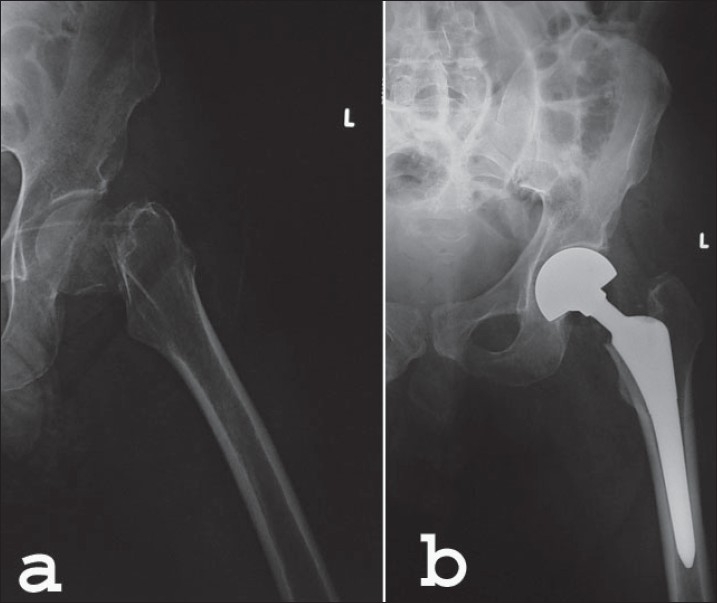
(a) Preoperative radiograph of a 88 year-old woman with a femoral neck fracture and (b) immediate postoperative X-ray of the same patient where cementless hemiarthroplasty is performed with the use of a porous-coated femoral stem and bipolar head. The fitting and filling of the medullary cavity is well visualized
